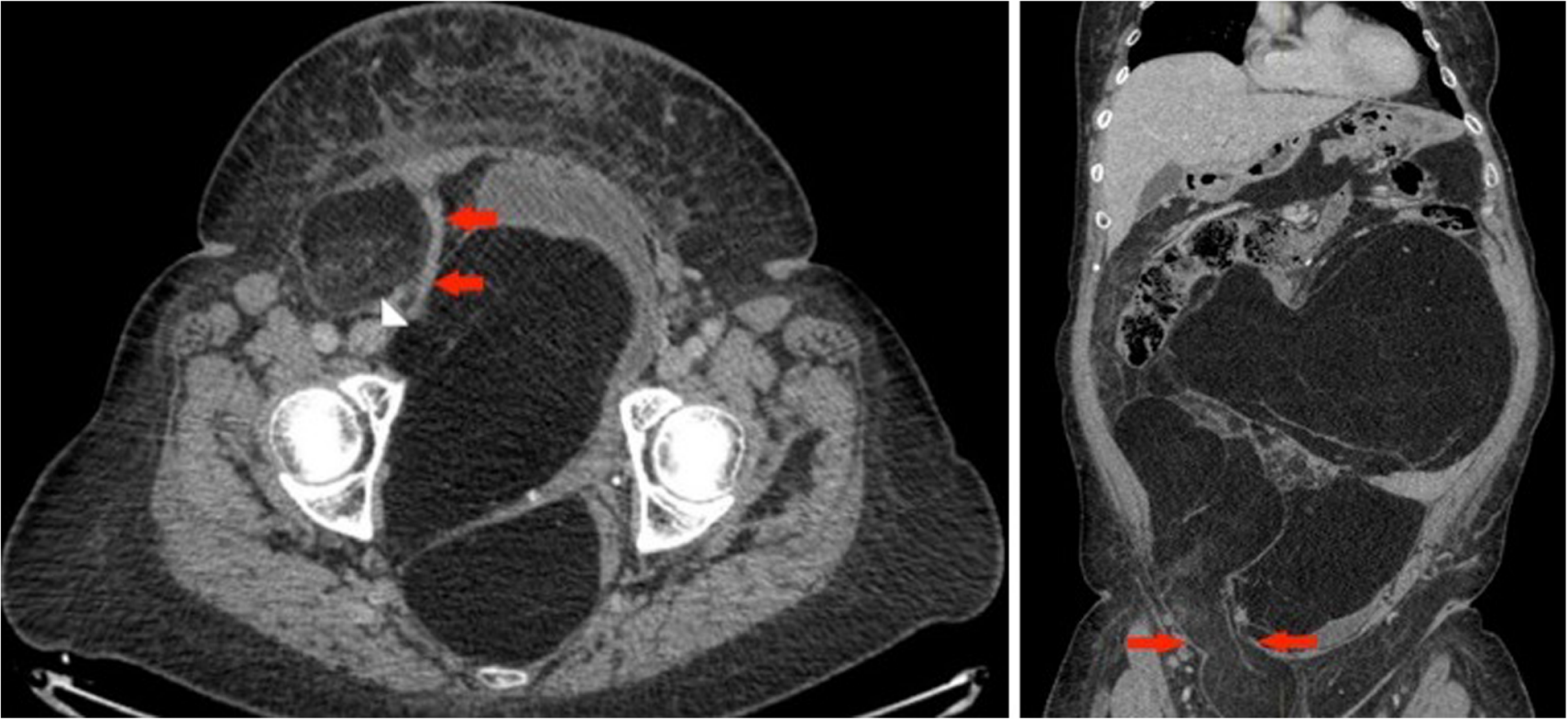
Left panel: Axial non-contrast-enhanced CT: a large pelvic mass predominantly of fat density is seen to compress and displace the bladder anteriorly. Part of the mass also extends into the right groin lateral to the epigastric vessels which are stretched over the lesion medially (red arrows). The white arrowhead points towards the origin of the inferior epigastric vessels from the external iliac vessels. Right panel: Coronal reconstruction of a non-contrast-enhanced CT, demonstrating the hernial neck (red arrows) as the tumour extends into the right inguinal canal
